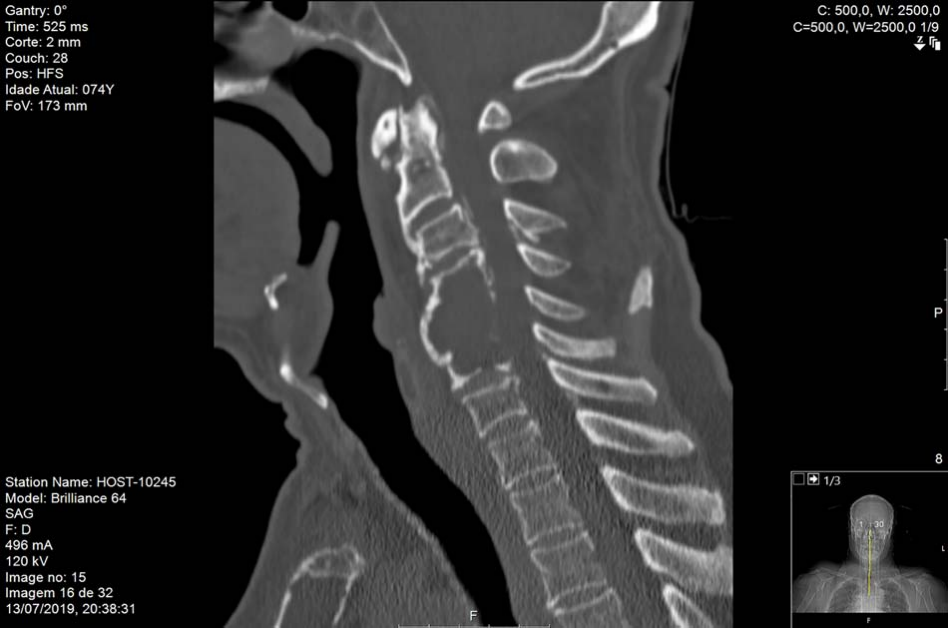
CT sagittal D120 post-RT.
